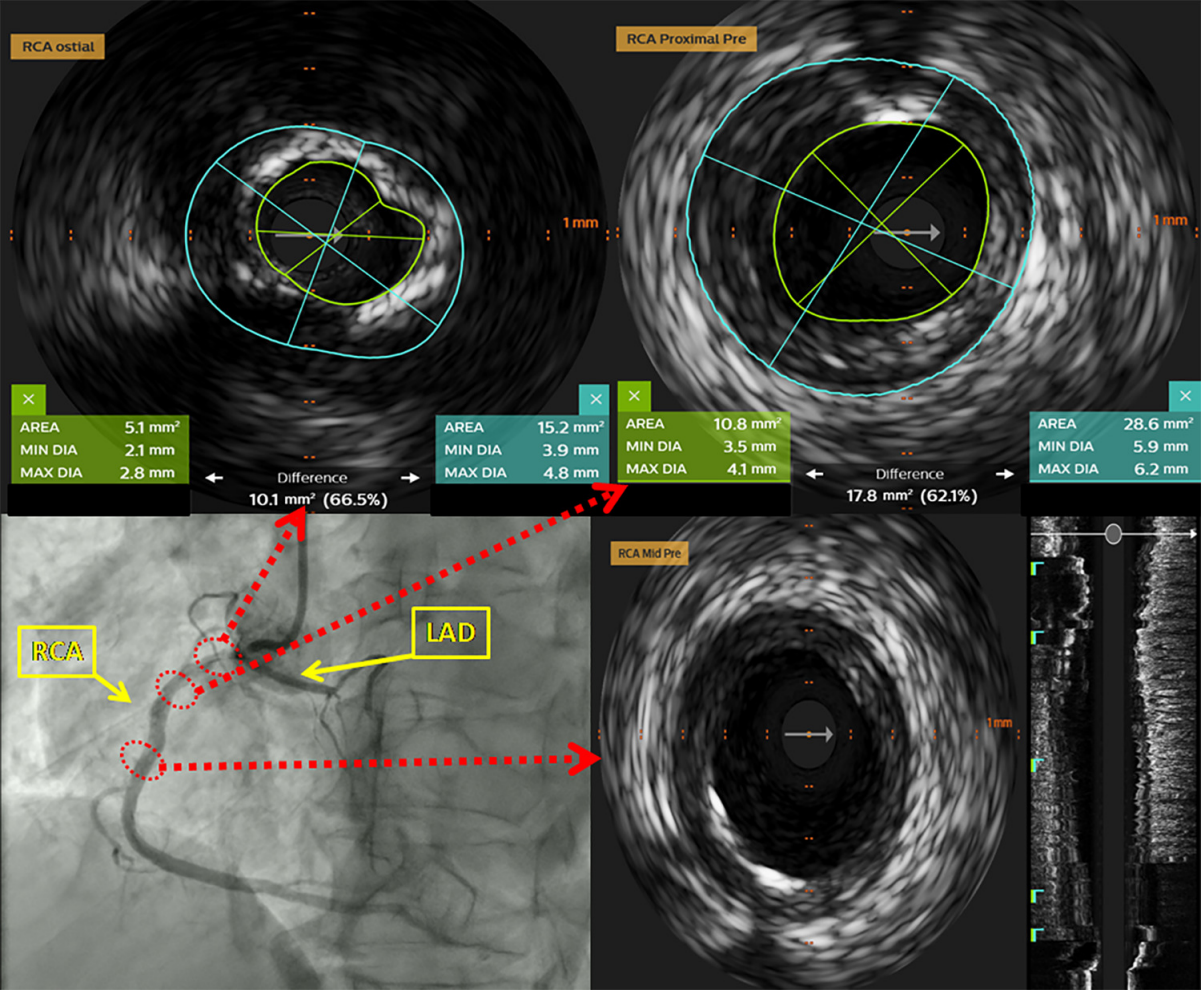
Intravascular ultrasound (IVUS) of the RCA prior to PCI. At the level of the ostial RCA segment highly calcified lesion was identified (more than 270° of vessel circumference). In the proximal segment of the RCA predominantly fibromuscular lesion was documented.
